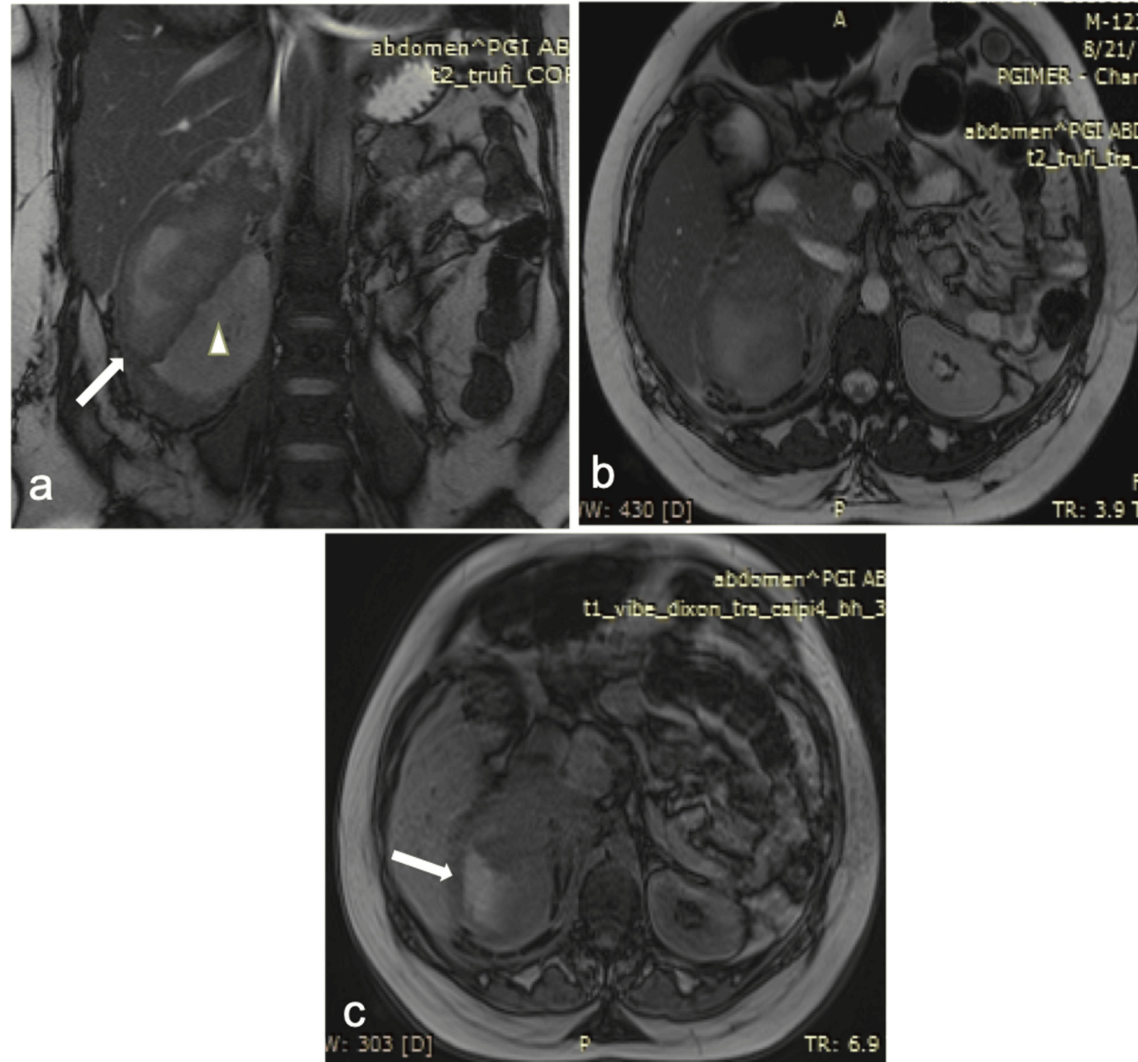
MRI findings. (a, b) Coronal and axial T2-weighted true fast imaging with steady-state free precession images show a heterogeneous, predominantly hyperintense lesion in the right suprarenal region (arrow). (c) Axial T1-weighted out-phase image showing hyperintense contents (arrow) within the mass lesion representing subacute hemorrhage. The right adrenal gland is not separately identified from the hematoma suggesting right adrenal hemorrhage. The right kidney is displaced inferomedially by the hematoma (arrowhead in a).
